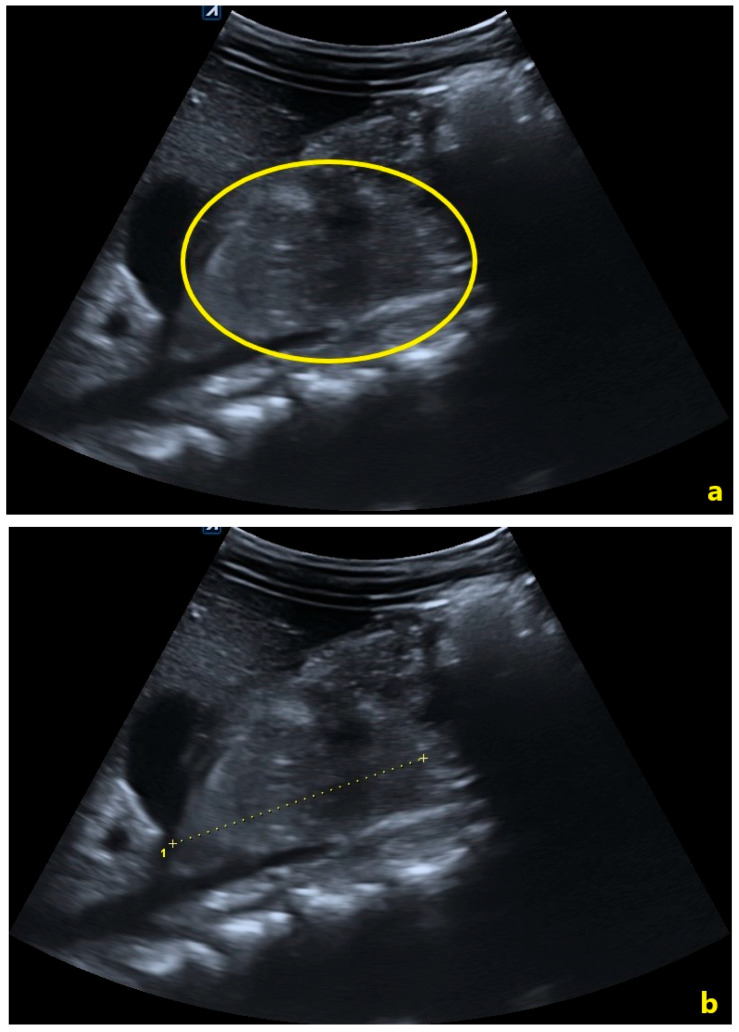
Abdominal ultrasound performed four hours post-injury. (a) The yellow oval delineates the heterogeneous hyperechoic pancreatic head, reflecting acute post-traumatic parenchymal changes. No organized fluid collection is identified at this stage. (b) Calipers demonstrate the craniocaudal dimension of the edematous pancreatic head, measuring approximately 57 mm.
